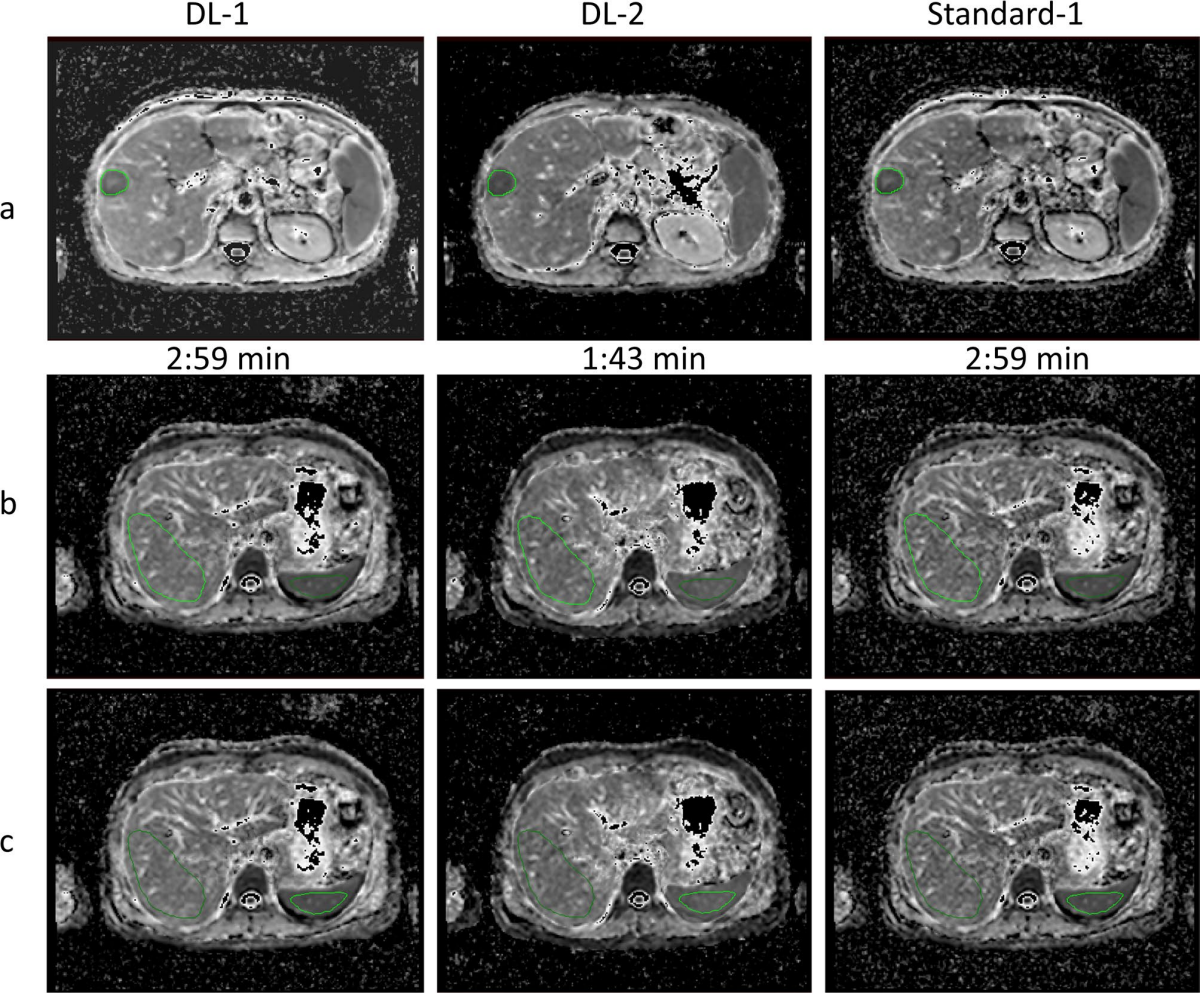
ROI delineation of the three anatomical locations from two different participants: a 40-year-old male participant (a) and a 47-year-old female participant (b, c) Liver metastasis (a), normal liver parenchyma (b), and spleen (c). For each patient, ROIs were matched across the three ADC maps derived from the two DWI acquisitions. Contrast display of all ADC maps was kept identical; ROIs for panel b and c are drawn on the same slice. ADC = apparent diffusion coefficient; DL-1 = moderately-accelerated DWI using a deep-learning reconstruction algorithm; DL-2 = highly-accelerated DWI using a deep-learning reconstruction algorithm; Standard-1 = moderately-accelerated DWI using a standard reconstruction algorithm
